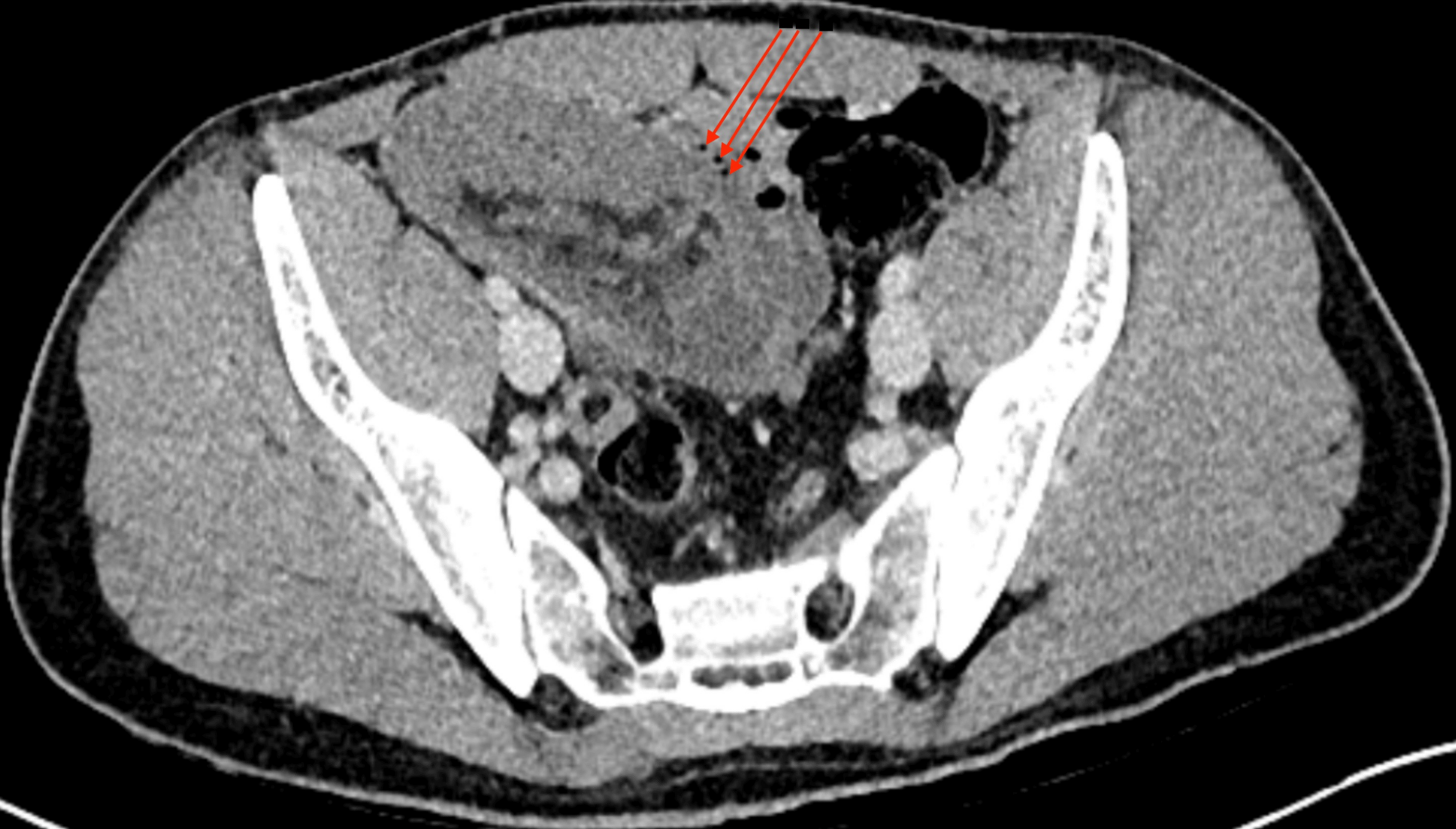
Ileocolic intussusception with hypoenhanced bowel with trace amount of extraluminal gas (red arrows). Axial view of CT abdomen and pelvis, portal-venous phase.
